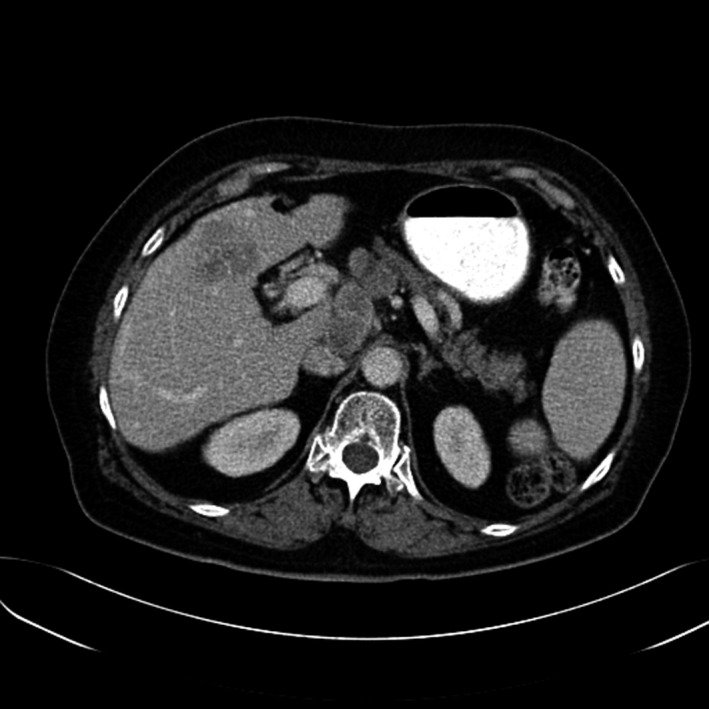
Patient 2. Baseline CT image portal venous phase.
